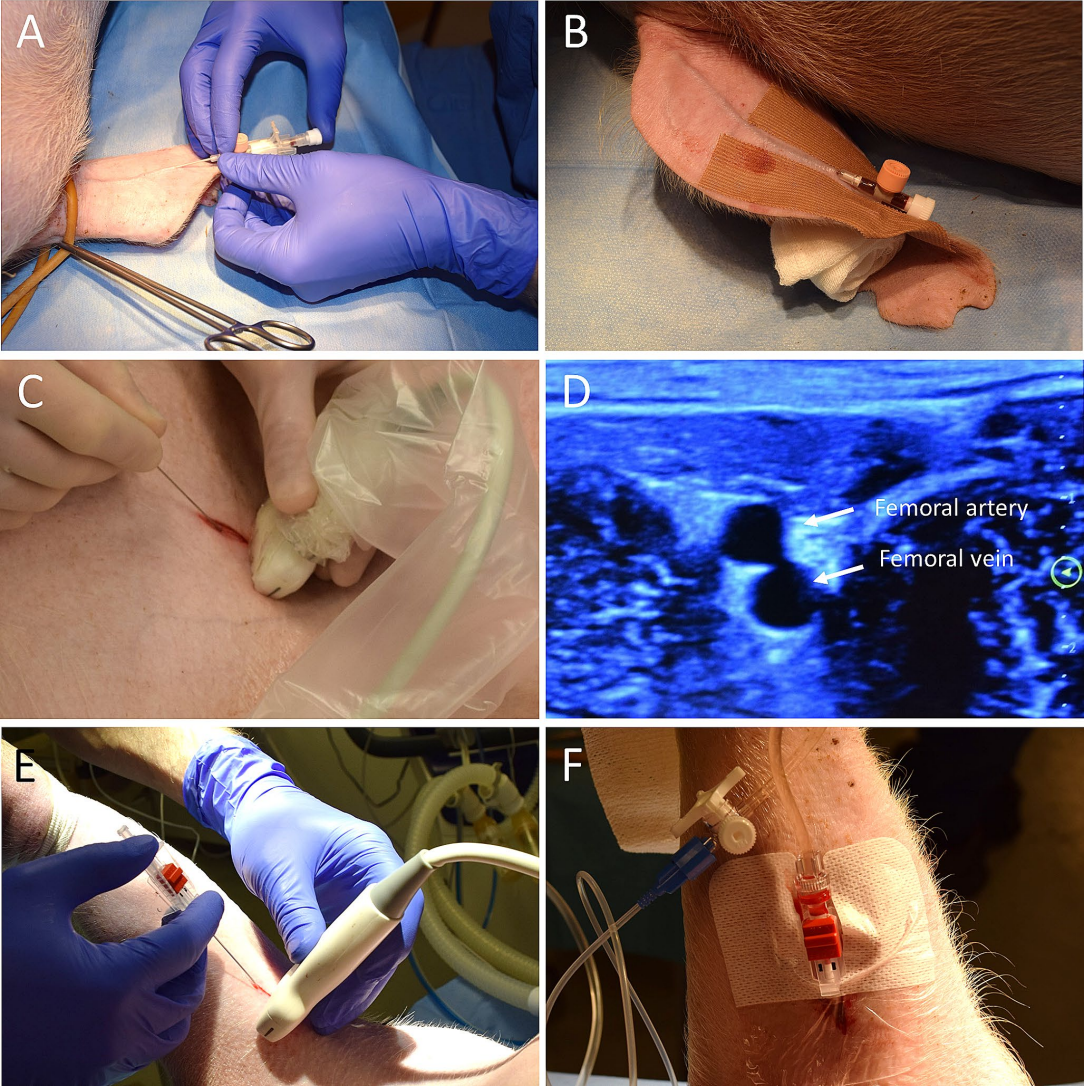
Vascular access. (A) The easiest venous access is obtained in the ear. (B) To keep the access open, place a rolled-up gauze in the ear to prevent the ear from folding. (C) Needle puncture for Seldinger technique access of the femoral artery through a skin incision perpendicular to the ultrasound probe. (D) Out of plane ultrasonogram of the femoral artery and femoral vein (scale in centimeters). (E) arterial needle is best placed in the foreleg, by first making a small incision and protruding the needle using ultrasound. (F) Keep the foreleg extended by traction to keep the arterial line open
